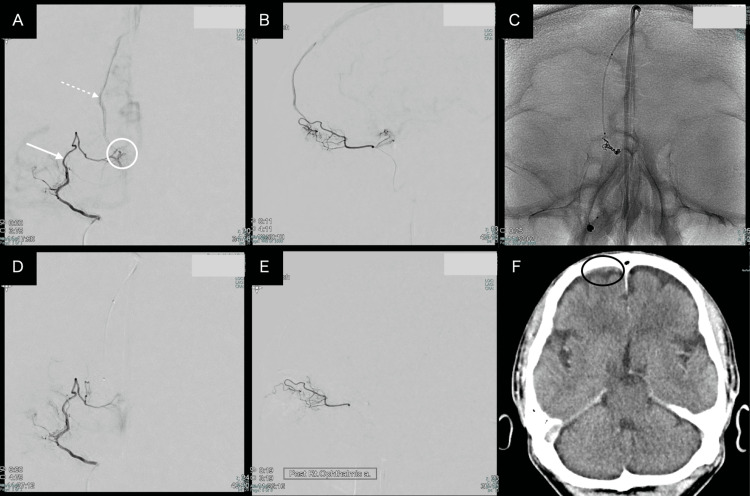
TVE of the second patient (A) Preoperative right ophthalmic artery angiography (white arrow) demonstrating the anterior ethmoidal artery supplying the shunt point (centered within the white circle), with a draining vein (white dashed line) visualized over the surface of the right frontal lobe. (B) Lateral view of right ophthalmic artery angiography. (C) Plain radiograph during TVE showing the microcatheter positioned at the shunt point with coil deployment in progress. (D) Postoperative anteroposterior view of right ophthalmic artery angiography demonstrating the complete obliteration of the shunt. (E) Postoperative lateral view of right ophthalmic artery angiography confirming the complete obliteration of the shunt. (F) Postoperative non-contrast CT demonstrating a small amount of hemorrhage over the surface of the right frontal lobe. TVE: transvenous embolization; CT: computed tomography
